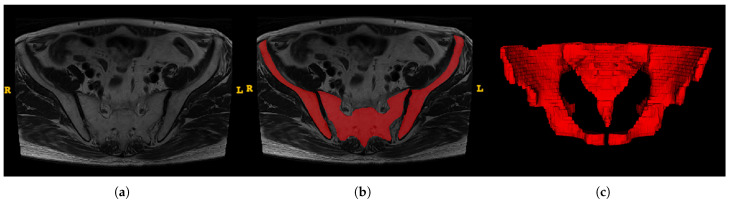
An example of manual segmentation of pelvis in MRI. (a) Bilateral ilium and sacrococcyx in T2-weighted images; (b) Manual segmentation on the same axial slice (Bilateral ilium and sacrococcyx are highlighted in red); (c) reconstruction of the pelvis.
